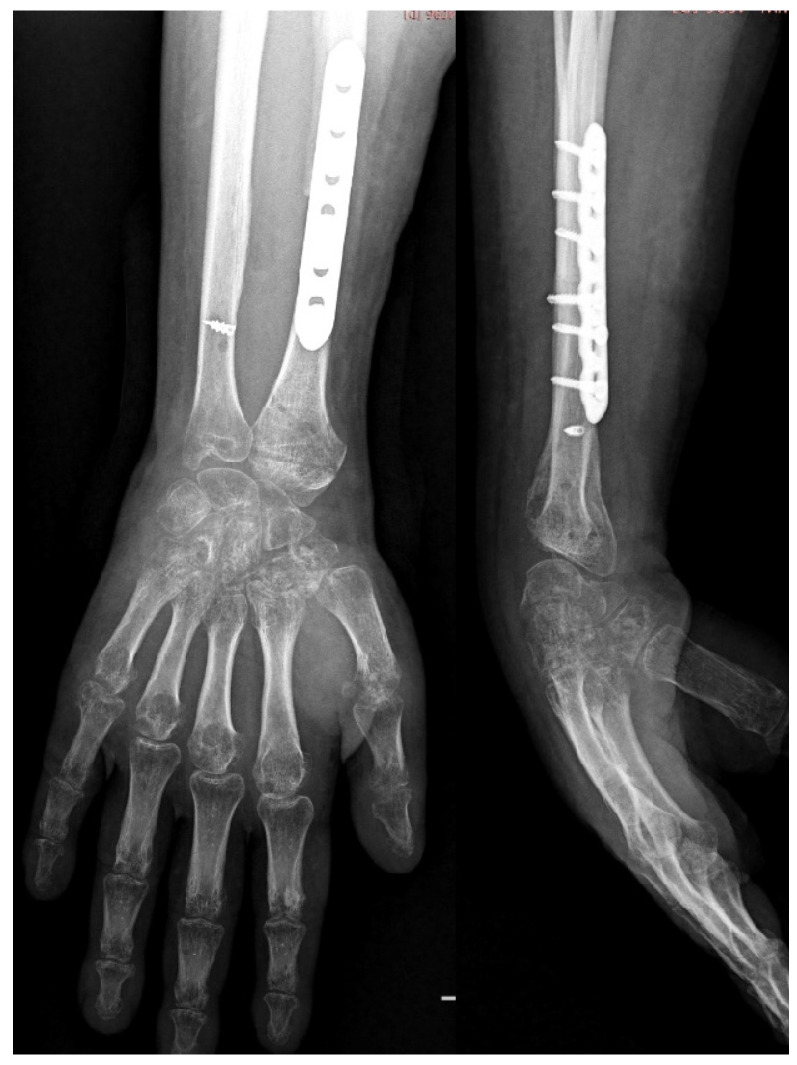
Three-month postoperative control radiographs (anteroposterior and lateral views) of the wrist joint, obtained after removal of the Kirschner wires.
